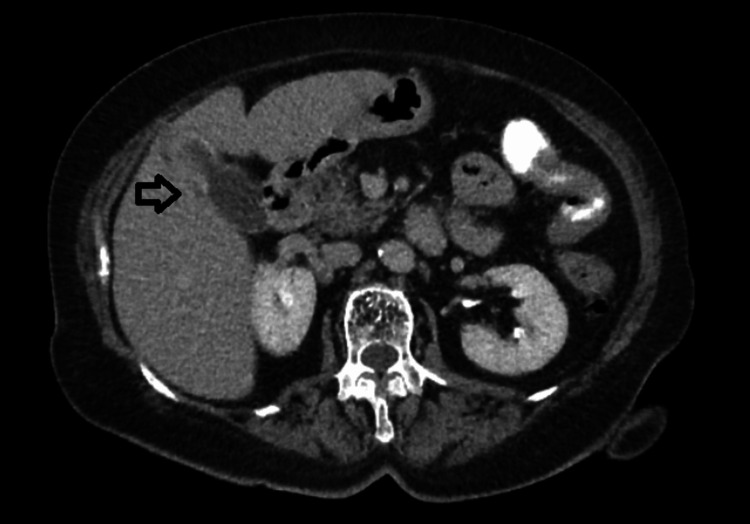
CT scan revealing a distended gallbladder with pericholecystic edema and a large gallstone
